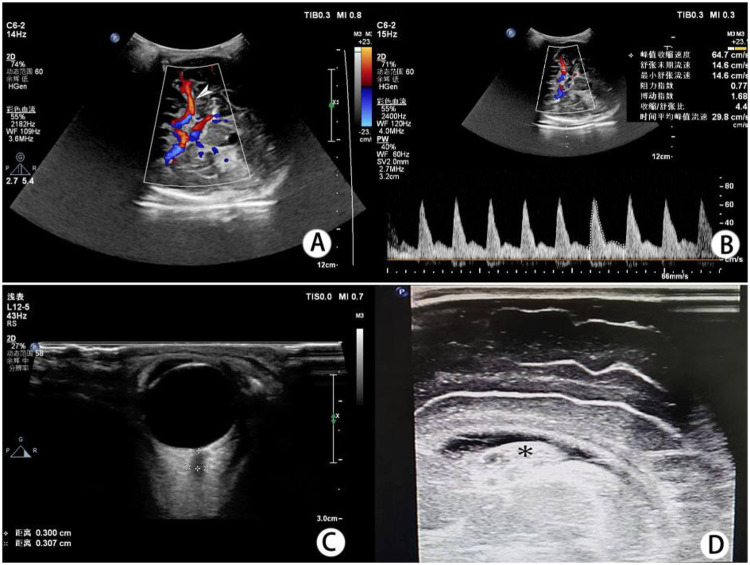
Bimodal ultrasound assessment and representative imaging findings. (A) Transfontanelle color Doppler ultrasound localization. The arrow indicates the M1 segment of the middle cerebral artery (MCA), which serves as the target vessel for functional assessment of cerebral hemodynamics. (B) Pulsed-wave Doppler spectrum of the middle cerebral artery. A stable cardiac cycle is demonstrated, allowing calculation of the pulsatility index (PI) and its coefficient of variation (PI-CV), which are used to quantify the resilience of cerebral autoregulation. (C) Measurement of optic nerve sheath diameter (ONSD). According to the CLOSED protocol, the diameter was measured 3 mm posterior to the globe (calipers shown), serving as a noninvasive surrogate marker of intracranial pressure. (D) Clinically significant IVH outcome phenotype. The neonatal sagittal section ultrasound showed grade II intraventricular hemorrhage, hyperechoic mass in the ventricle (indicated by asterisk) and normal ventricular size.
